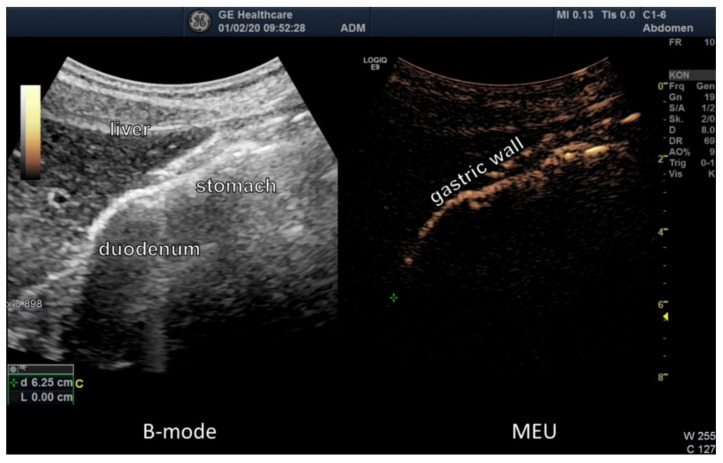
Ultrasound image of the stomach. B-mode after ingestion of the mixture with vitamin C, the substance was immediately detected as an echo amplification by ultrasound in the stomach. The microbubbles (MEU) migrated to the stomach wall and are clearly visible.
